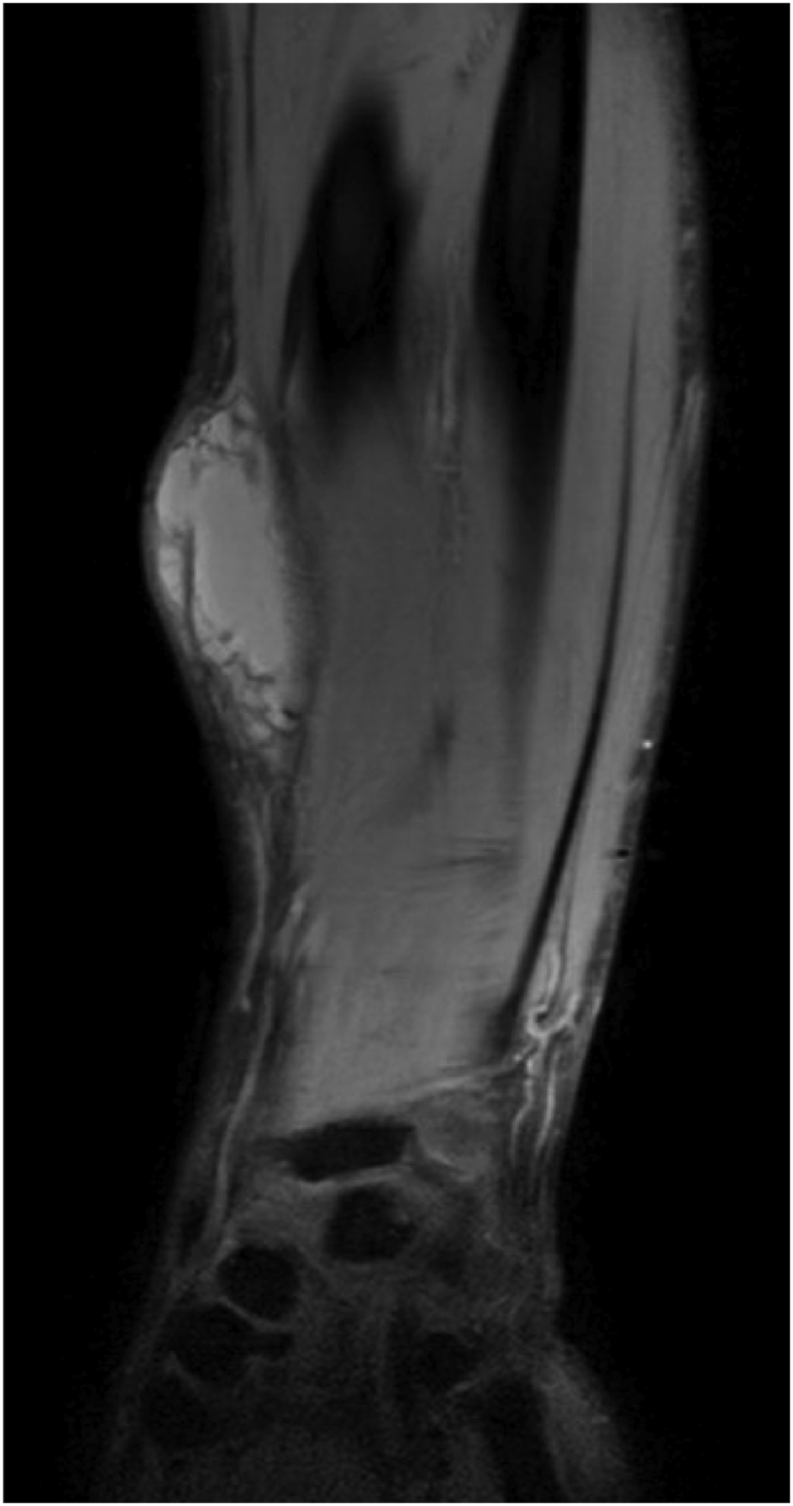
Case 2: Lesion of the right forearm on MRI (day +3).
